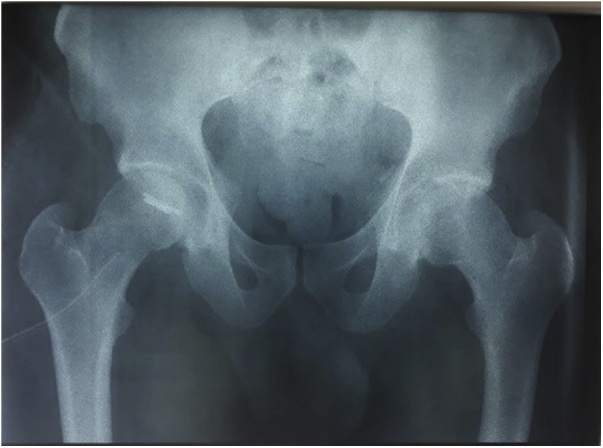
pelvic post-operative X-ray showing the Herbert screws fixation and good anatomic hip reduction.
